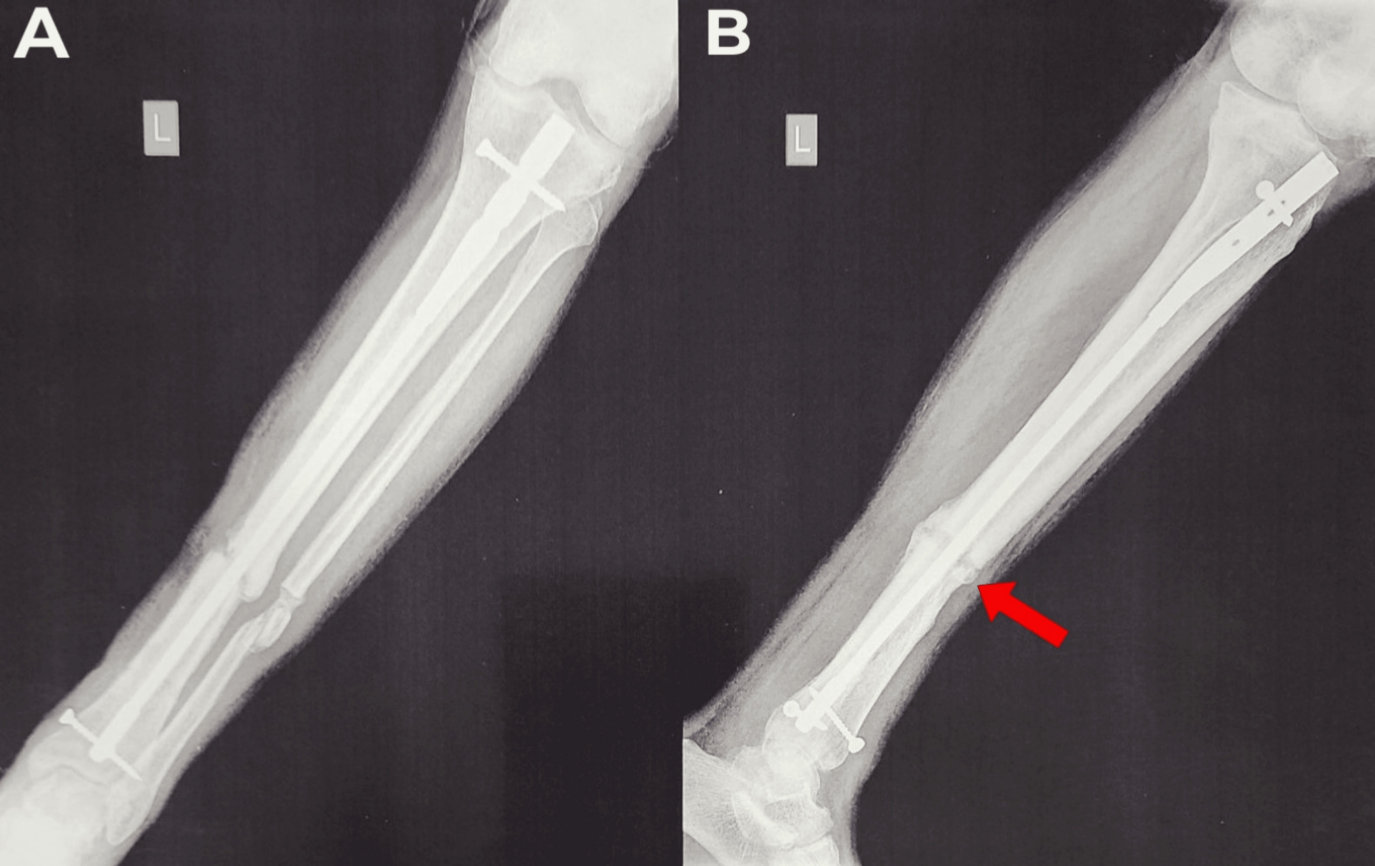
A) Left tibia and fibula full-length AP X-ray with good callus formation. B) Left tibia and fibula full-length lateral X-ray with increased callus deposit in anterior cortex region of the non-union site. A red arrow shows an increased callus deposit in the anterior cortex region of the non-union site. AP: anterior posterior
